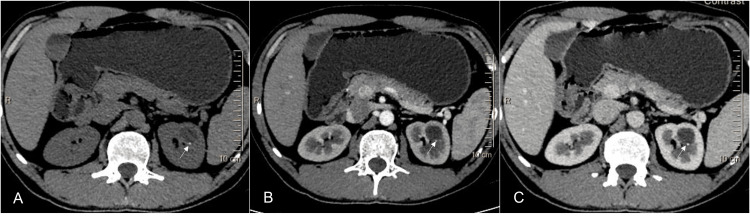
A 40-year-old female with an incidentally detected left renal cyst Axial NCCT (A), CECT corticomedullary phase (B), and nephrographic phase (C) images show a cyst with homogenous fluid density in the left kidney, with an eccentric speck of calcification along its posterior wall (white arrow). The cyst wall is imperceptible and non-enhancing. This represents a Bosniak II cyst. NCCT: non-contrast-enhanced CT; CECT: contrast-enhanced CT
